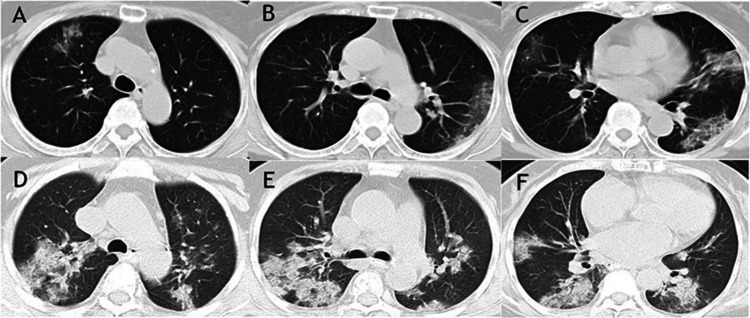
Pulmonary changes with progression of the disease A-C: stages of the disease that resulted in resolution. D-F: CT images of a patient who succumbed to the disease. Image source: Reference no. [16]
